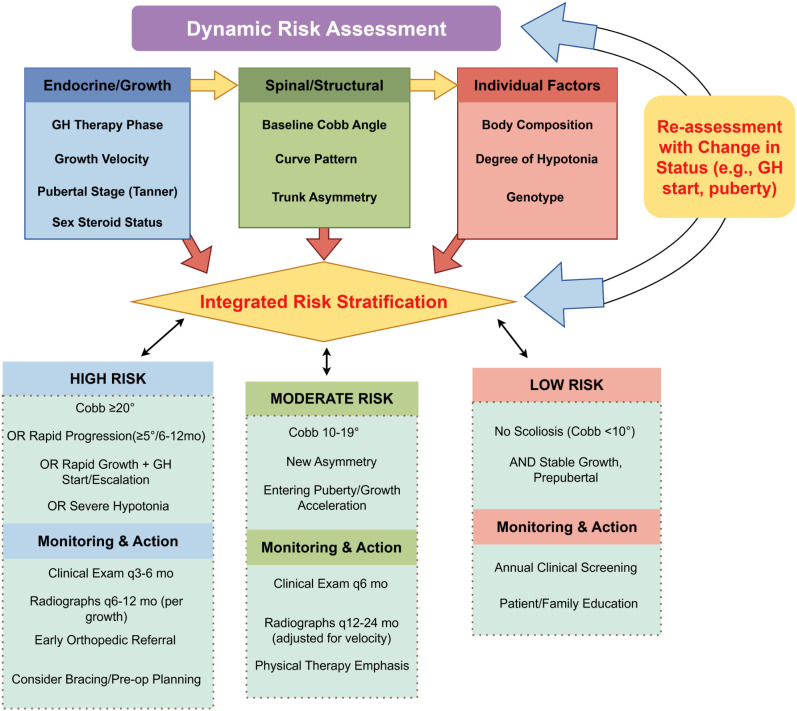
An endocrine-informed, risk-stratified framework for scoliosis monitoring in Prader-Willi syndrome. This clinical decision algorithm proposes a dynamic monitoring framework. It integrates inputs from endocrine/growth status, spinal parameters, and individual factors to perform continuous risk stratification. Patients are categorized into low, moderate, or high-risk states, which dictate specific recommendations for surveillance intensity (clinical and radiographic follow-up frequency) and clinical actions (e.g., physical therapy emphasis, orthopedic referral). The circular arrow emphasizes the need for re-assessment whenever key status changes (e.g., GH initiation, puberty onset), ensuring monitoring aligns with individual developmental trajectories. Proposed framework requiring clinical validation. GH, growth hormone; Cobb, Cobb angle; mo, months. Image drawn by www.figdraw.com. Crucially, the risk thresholds, particularly the Cobb angle criteria, must be interpreted within the context of age of onset. For early-onset scoliosis (onset <10 years), progression thresholds are more aggressive and should be guided by the EOS-C system and evaluated by a specialist, rather than relying solely on the >20° threshold indicated here, which is primarily informative for adolescent-onset curves.
